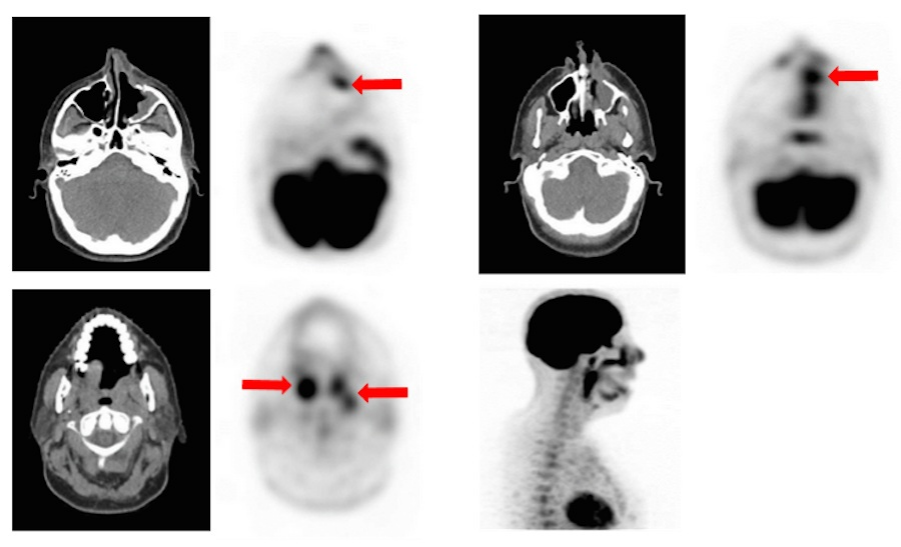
Restaging FDG-PET/CT PET-CT revealing FDG-avid lesions in the anteromedial left​ maxillary sinus surgical bed, as well as FDG-avid right adenoid tonsillar lesion, squamous temporal bone involvement, and left parapharyngeal nodes (see arrows).
